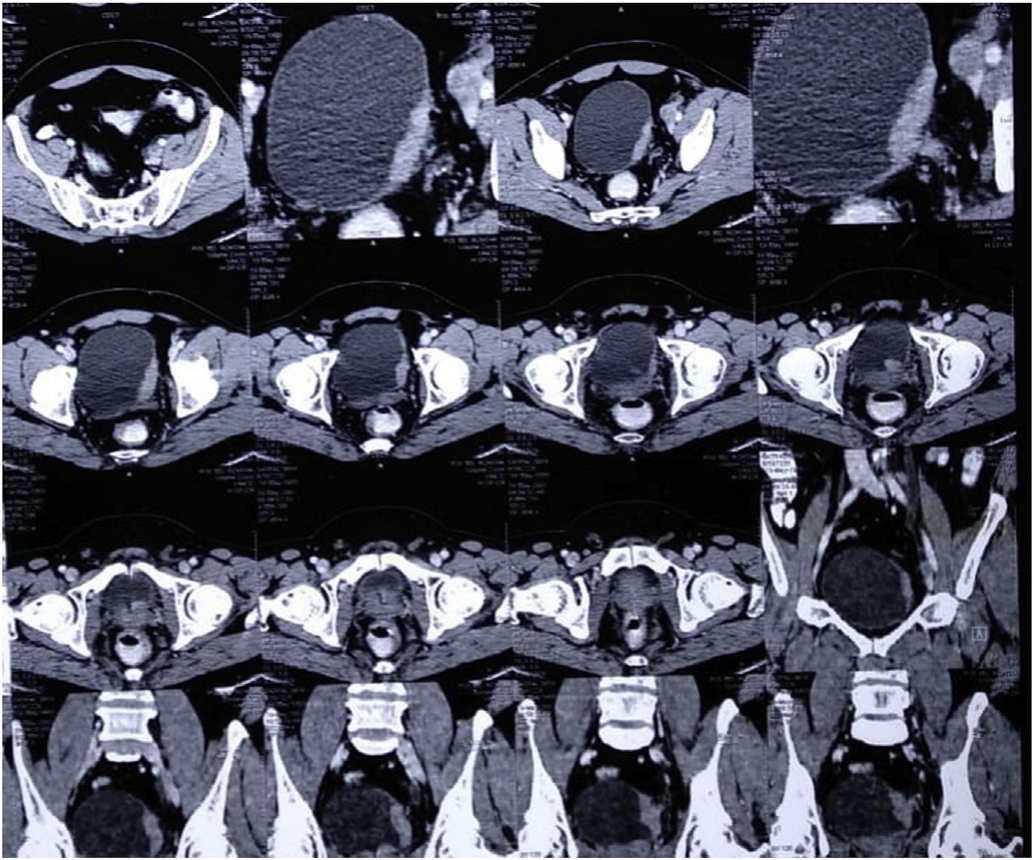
On CT Scan, diffuse thicking of urinary bladder is evident.
